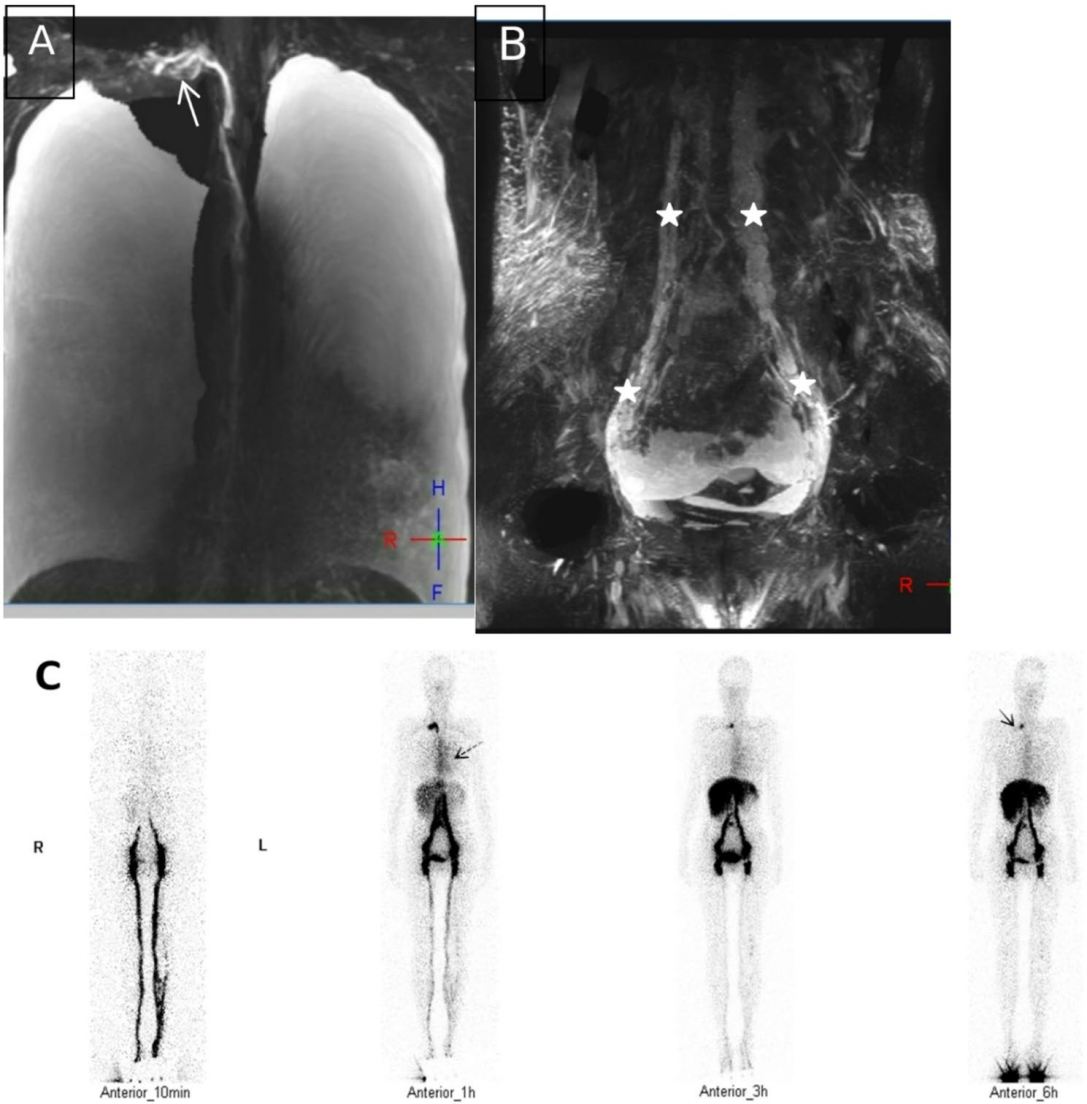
A–C A patient with SLE with lymphatic system involvement. (A) Coronary NCMRL showing drainage of the thoracic duct to the right jugular venous angle (white arrow). (B) Coronary NCMRL showing widened T2-weighted hyperintensities (pentagrams) around the bilateral retroperitoneal large vessels and iliac vessels. (C) ^99^TC^m^-DX lymphoscintigraphy (anterior): right jugular venous angle is continuously visible at 1 h, 3 h, and 6 h (black arrow); elevated radioactivity in the left chest cavity (dashed black arrow) indicates left chylous pleural effusion
